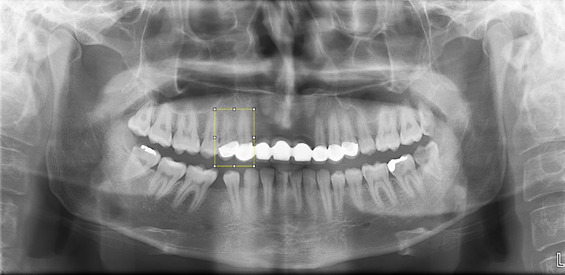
Cropped panoramic radiographs showing zirconia-based fixed restoration and metal-supported porcelain fixed restoration. The yellow box indicates the selected ROI.
